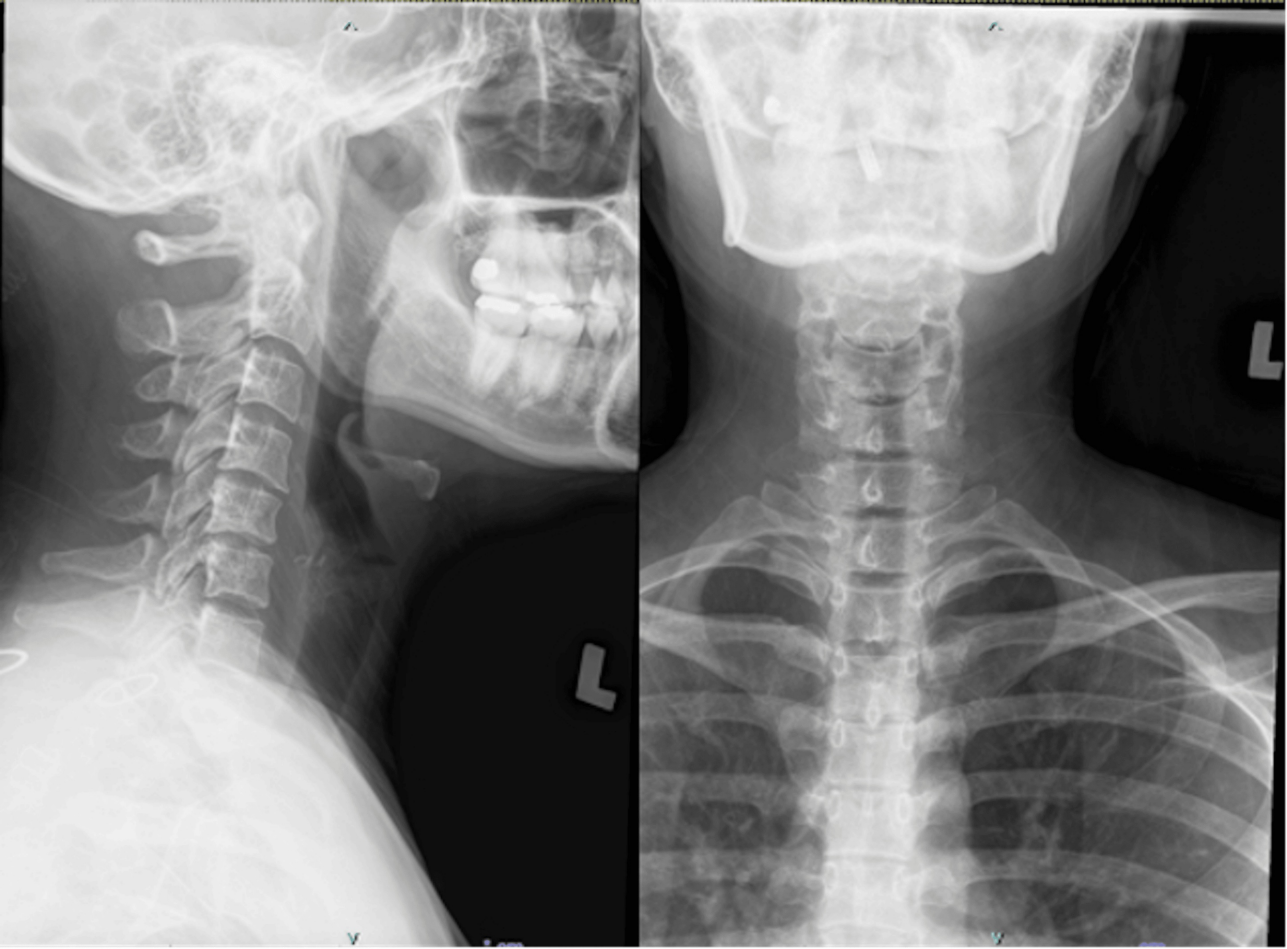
Plain radiographs of the cervical spine in lateral and anterior-posterior views showed reduced joint space at the C4/C5 and C5/C6 levels.
